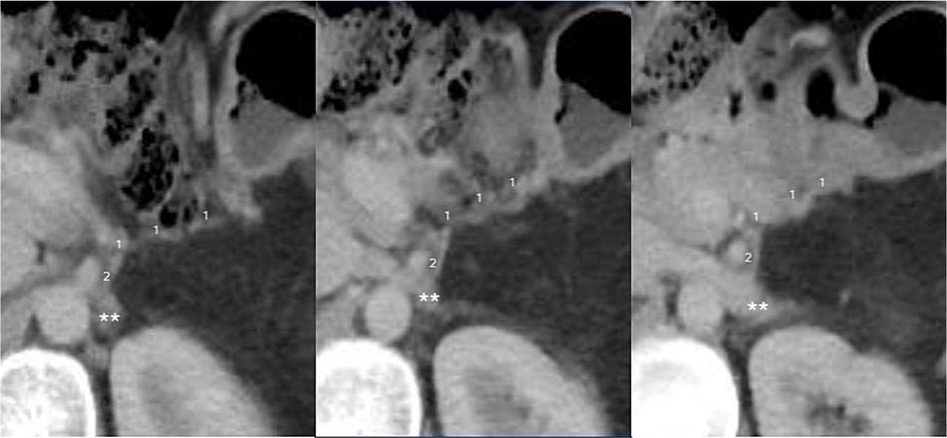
CT scan taken 12 months after surgery, displayed from upper to lower in a left to right sequence, showing the anastomosis between the left gastric vein (LGV, 1) and left adrenal vein (LAV, 2). The left renal vein is indicated by ‘^^’.
